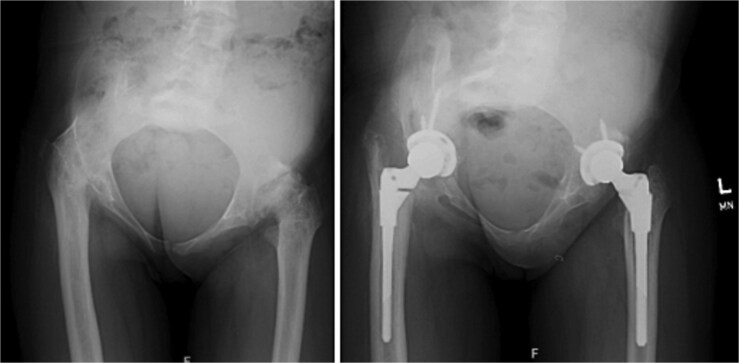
Anteroposterior radiographs of the pelvis demonstrating bilateral hip ankylosis prior to surgical intervention (left) and following staged bilateral total hip arthroplasty with restoration of joint alignment (right).
